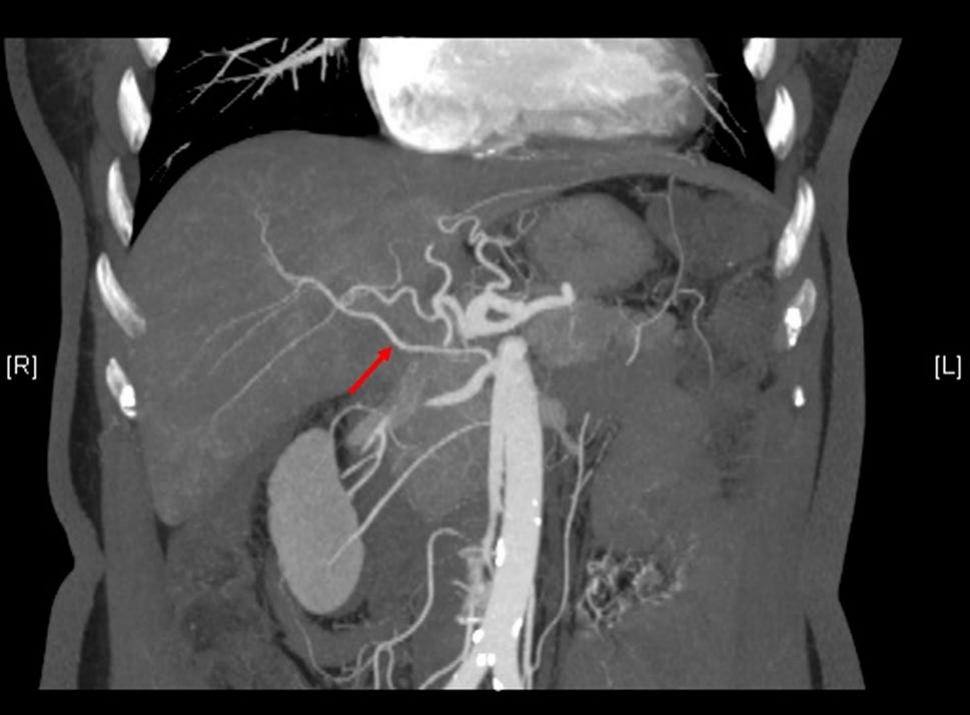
Maximum intensity projection of enhanced CT scan shows that the aRHA, derived from the SMA, ran into the RAL. RPHA was derived from the PHA
